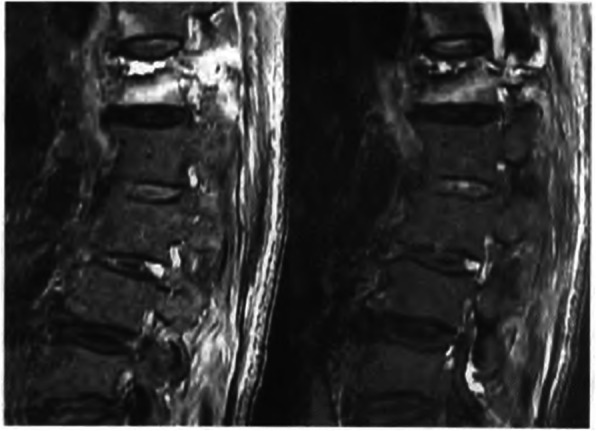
Preoperative MRI film of L_1_ vertebral compression fracture
